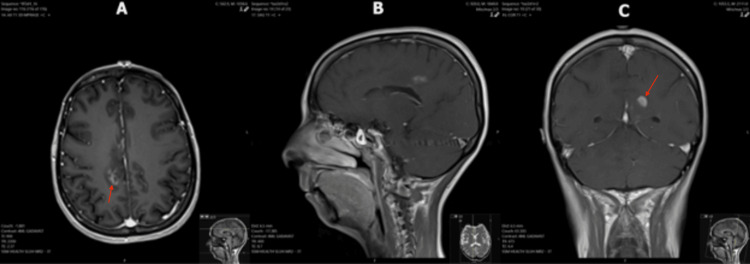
T1 post-contrast MRI images demonstrating significant interval regression of multiple PCNSL lesions after steroid administration (A) Axial image demonstrates an interval decrease in size of the right frontoparietal/cingulate gyrus lesion (arrow) and corresponding reductions in vasogenic edema and mass effect. (B) Sagittal image shows near-complete resolution of the cingulate gyrus, corpus callosum, basal ganglia, and hypothalamus lesions. (C) Coronal image with a small but enlarged lesion in the left cingulate gyrus (arrow). There was complete interval resolution of the previously noted enhancing lesions adjacent to the bilateral occipital horns of the lateral ventricles. MRI: magnetic resonance imaging; PCNSL: primary central nervous system lymphoma
